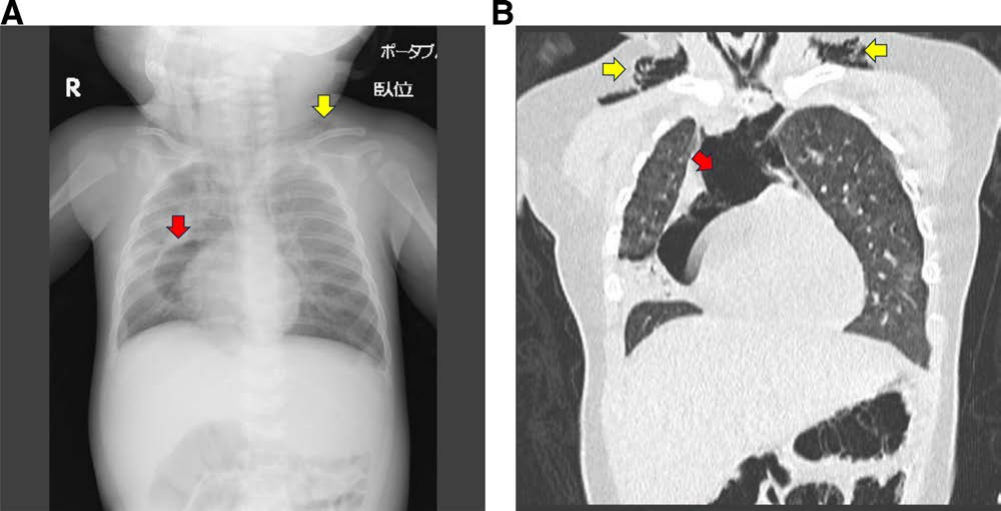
(A) Chest X-ray shows pneumomediastinum (red arrow) and subcutaneous emphysema (yellow arrow). (B) Coronal CT image shows severe pneumomediastinum (red arrow) and subcutaneous emphysema (yellow arrows). CT = computed tomography.
